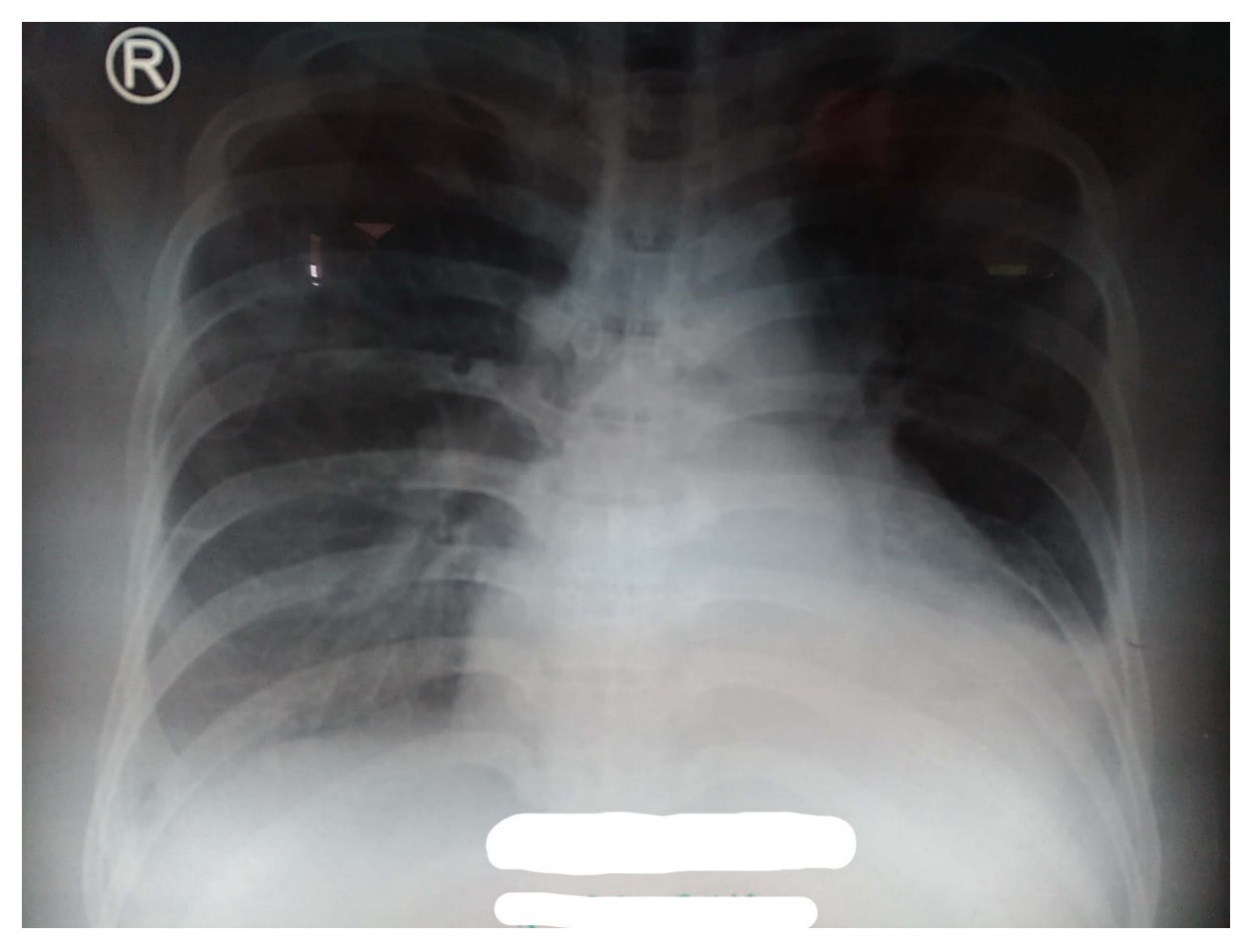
Chest X-ray of the patient.
